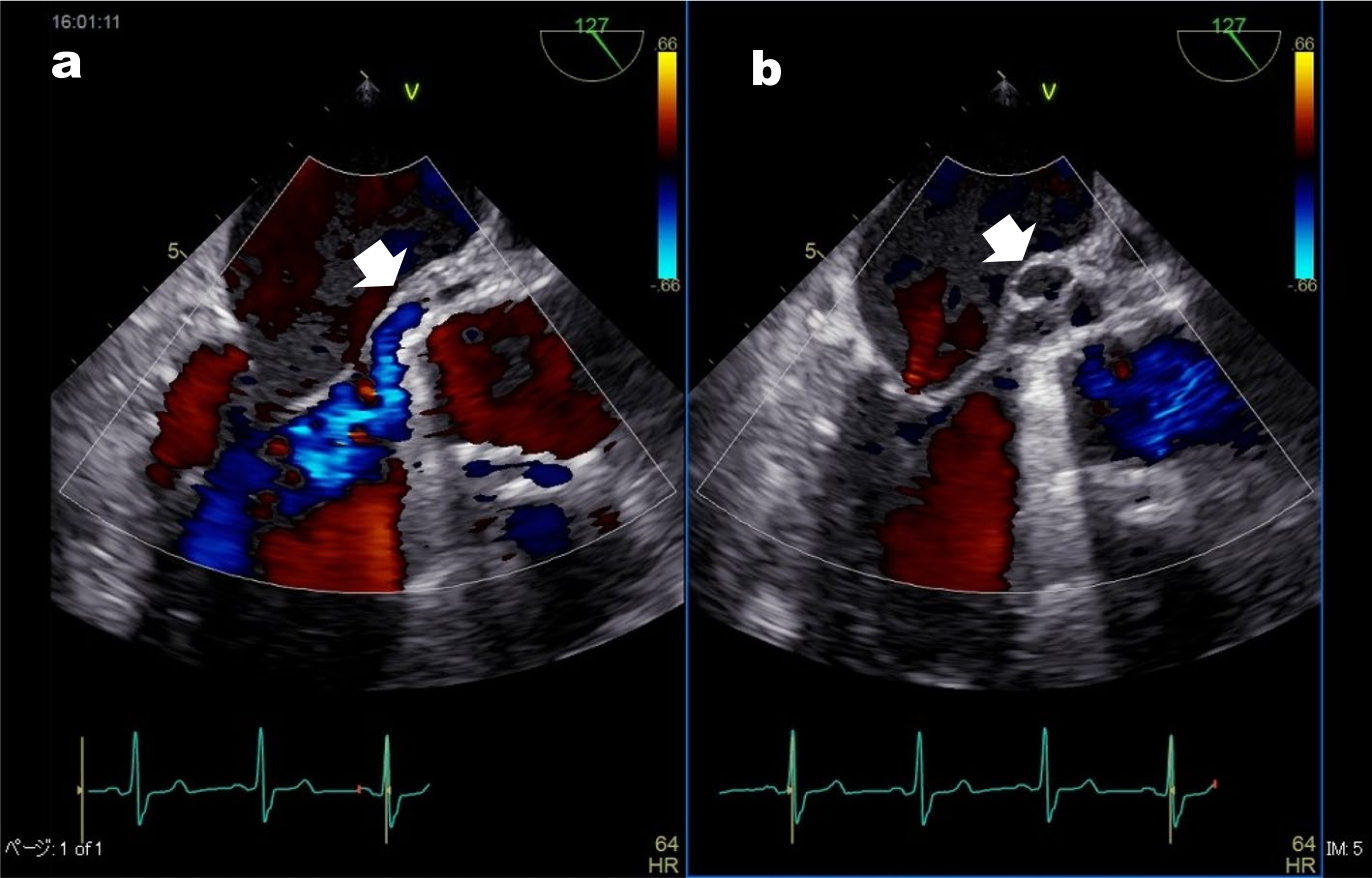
Transesophageal echocardiography (TEE). TEE showed a 13.4-mm × 23.6 mm × 12.1 mm pseudoaneurysm with a septum posterior to the aortic valve and blood flow communicating with the pseudoaneurysm and left ventricle, a characteristic finding of P-MAIVF with systolic expansion (b, arrow) and diastolic collapse (a, arrow). There was blood flow entering the P-MAIVF during systole and leaving from the P-MAIVF to the LVOT during diastole
